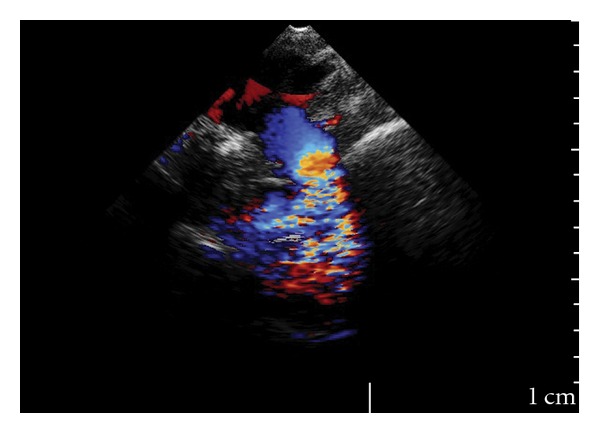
Transthoracic suprasternal view with a turbulent flow below the origin of subclavian artery.
